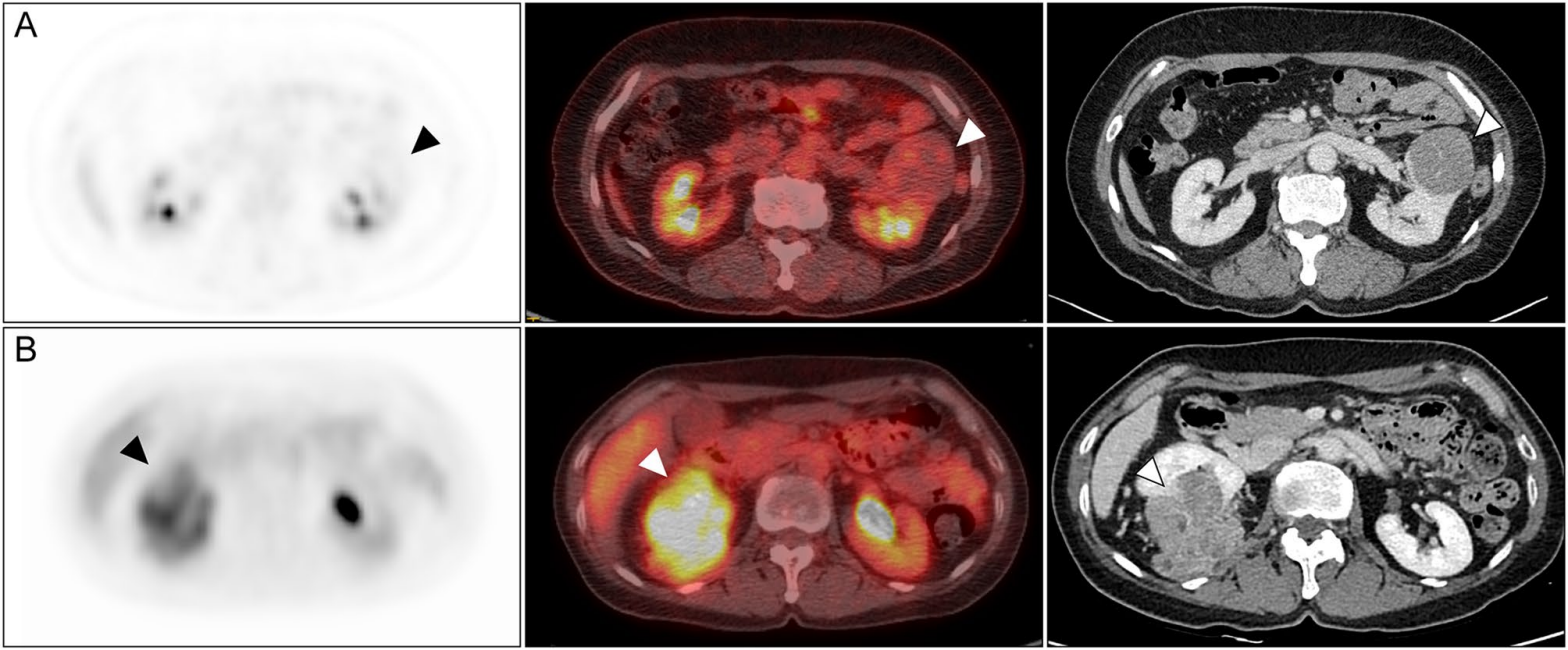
RCC with low and high MTV. Upper row: a 66-year-old female had a renal mass (arrowhead) with low ^18^F-FDG uptake (SUV_max_: 2.98, MTV: 1.44 cm^3^). The pathological T stage was T1b. Lower row: a 63-year-old female had a renal mass (arrowhead) with high ^18^F-FDG uptake (SUV_max_: 6.85, MTV: 99.16 cm^3^). The pathological T stage was T3a. *RCC* renal cell carcinoma, *FDG* fluorodeoxyglucose, *SUV*_*max*_ maximum standardised uptake value, *MTV* metabolic tumour volume.
